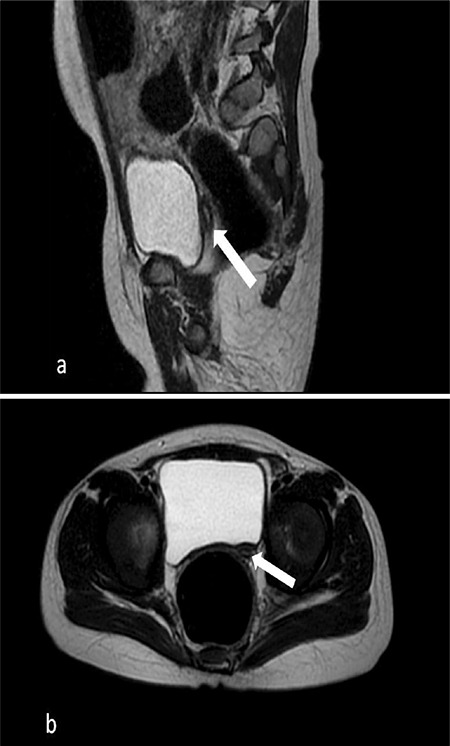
Rudimentaty uterus is shown on the sagittal T2 (a) and axial (b) T2-weighted magnetic resonance images
